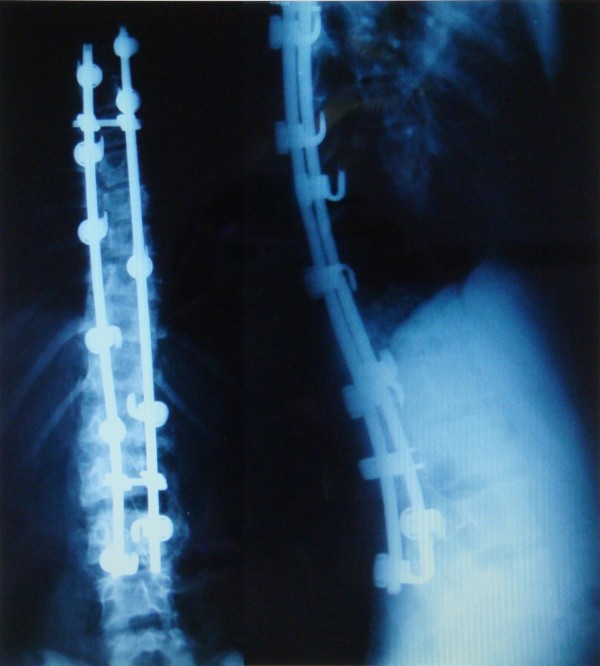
Postoperative radiographs of the patient on Figure 1. Posteroanterior and lateral standing views 5 years after surgery. The patient was completely asymptomatic at the latest follow-up visit.
